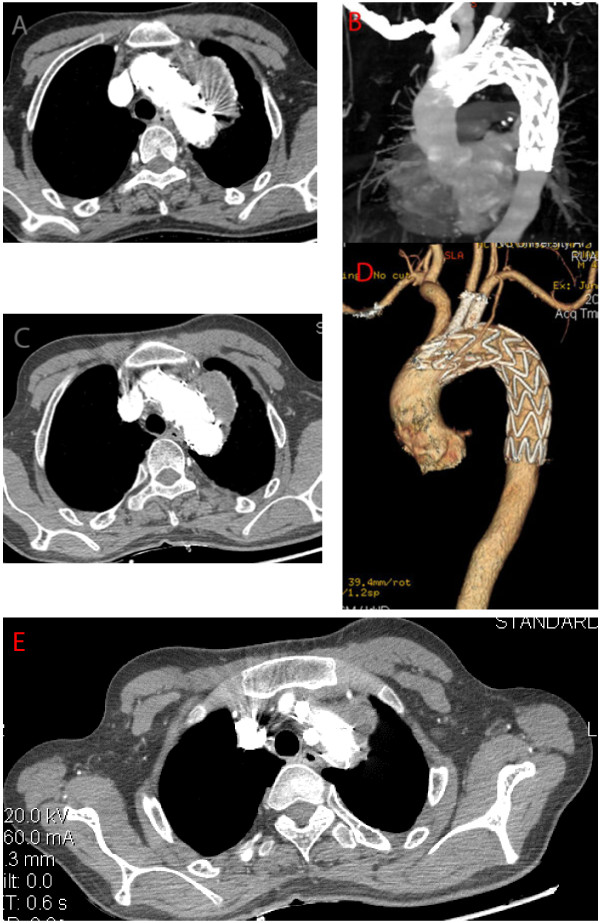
Follow-up CTA: CTA on the first week post-operative follow-up showed no evidence of proximal endoleak, no filling or enlargement of the pseudoaneurysm (A,B). Postoperative CTA demonstrated thrombus formation in the pseudoaneurysm lumen, no endoleak and the aortic arch, LCCA and LSA were patent in 12-month follow-up (C,D,E).
